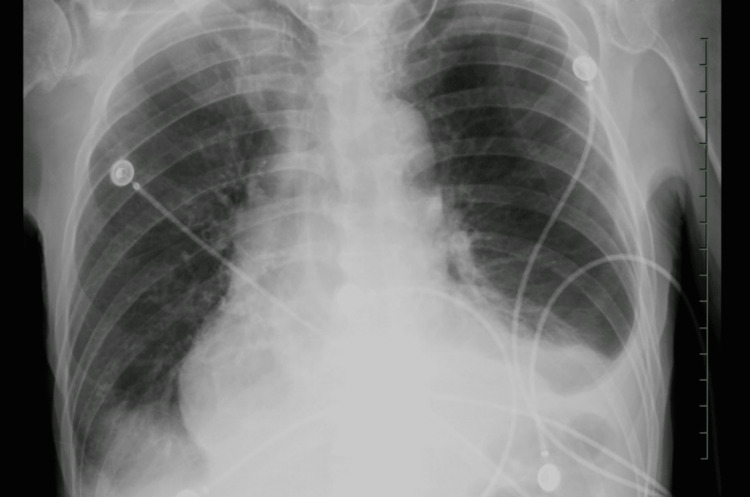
Chest x-Ray showing bibasilar hazy airspace opacities
